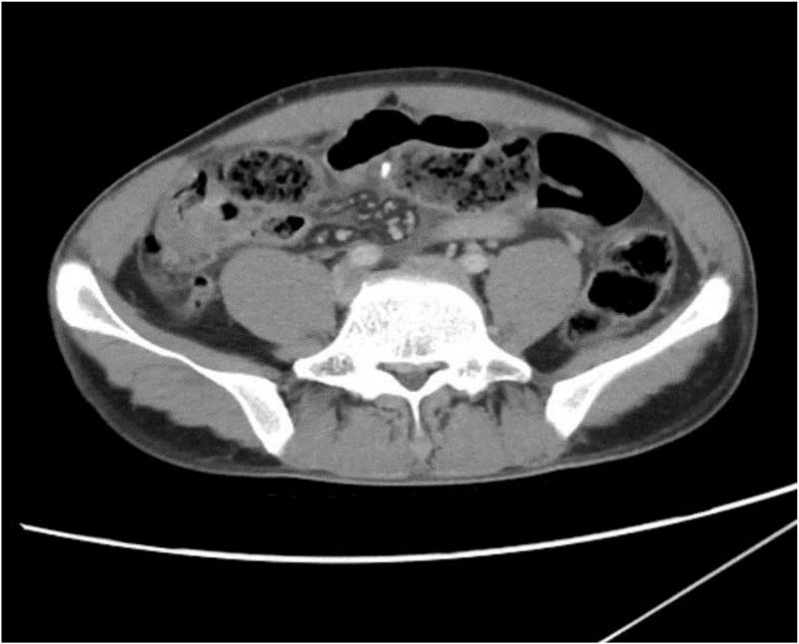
CT scan of abdomen and pelvis. A radiopaque object is seen extruding from a thickened small bowel loop with associated free peritoneal fluid, concerning for foreign body perforation of small bowel.
